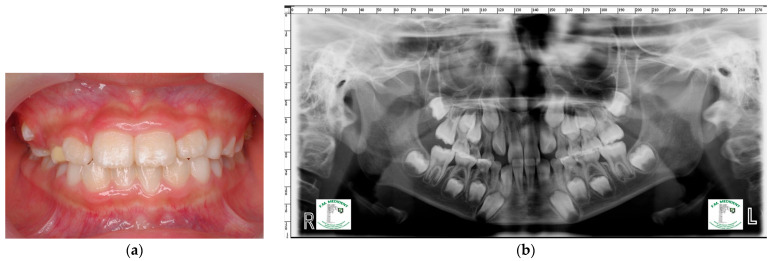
(a) Patient’s frontal occlusion one week prior to trauma; (b) panoramic X-ray one year prior to trauma, March 2018.
